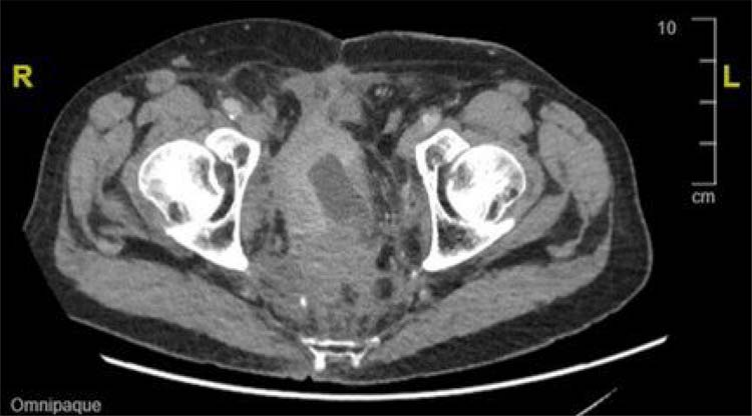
CT image showing an extensive mass in the presacral region with invasion to the urinary bladder and both ureters.
